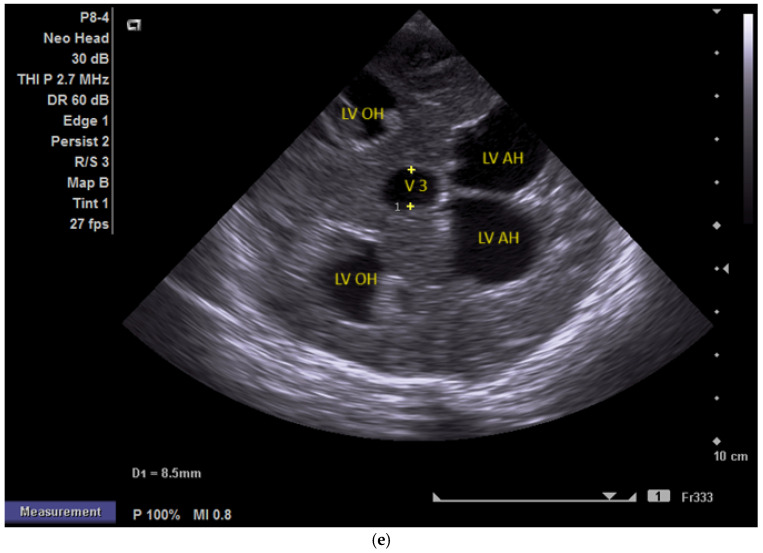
(a,b) MRI—T2 sequence. Sagittal view. Antenatal. Yellow arrows indicate the place of the suspected tumor (personal image collection). (c) Cranial ultrasound. Sagittal view. Up—enlarged left ventricle. D1—thamalo-occipital distance. Down—enlarged right ventricle. D1—thalamo-occipital distance (personal image collection). (d) Cranial ultrasound. Sagittal view. Enlarged third ventricle. Fourth ventricle is normal. D1, D2—measurements of the third ventricle (personal image collection). (e) Cranial ultrasound. Transtemporal view. Enlarged third ventricle. Sylvian aqueduct is not visible. D1—measurement of third ventricle. LV AH—lateral ventricle anterior horn. LV OH—lateral ventricle occipital horn. V 3—third ventricle (personal image collection).
